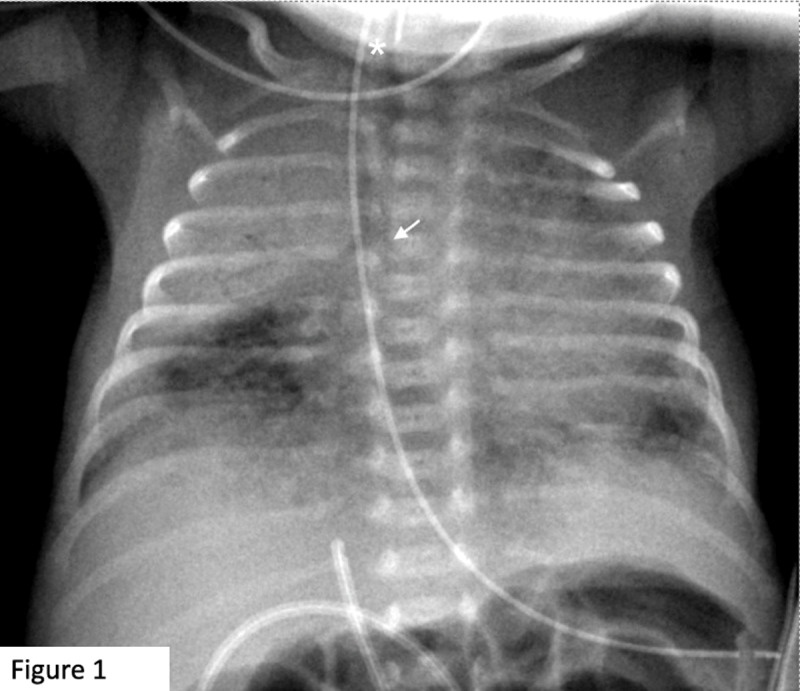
Following axial computed tomography (CT) angiography (Figure 2A), an aberrant origin of the left pulmonary artery (LPA) from the right pulmonary artery (RPA) was seen, with a subsequent posterior compression of the trachea on sagittal reformation (Figure 2B, arrow), causing a tight stenosis of the pre-carinal trachea. The carina is located at the level of the seventh thoracic vertebral body. Coronal reformation (Figure 2C) shows an abnormal origin of the right upper lobe bronchus on the right, with abnormal narrowing of the tracheal segment below this origin, due to the abnormal origin and course of the LPA.
